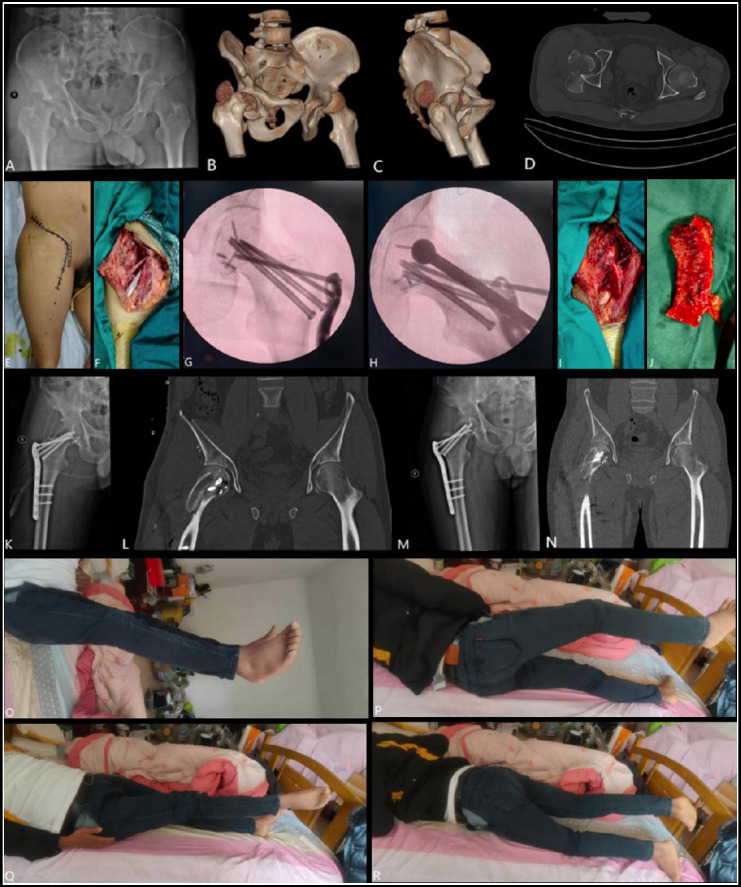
A thirty-seven-year-old male with right Pipkin Type-III fracture. (A) Preoperative X-ray. (B-D) Preoperative CT showing comminuted fractures. (E-F) Intraoperative SP approach. (G) Screw and locking plate fixation. (H) Femoral neck slotting and bone flap insertion. (I-J) Bone flap dissection showing bright red blood flow. (K) Postoperative X-ray. (L) Postoperative CT showing good reduction. (M) Two-year follow-up X-ray. (N) Two-year follow-up CT showing no necrosis and good healing. (O-R) Postoperative hip function showing good flexion, abduction, external rotation, and extension.
